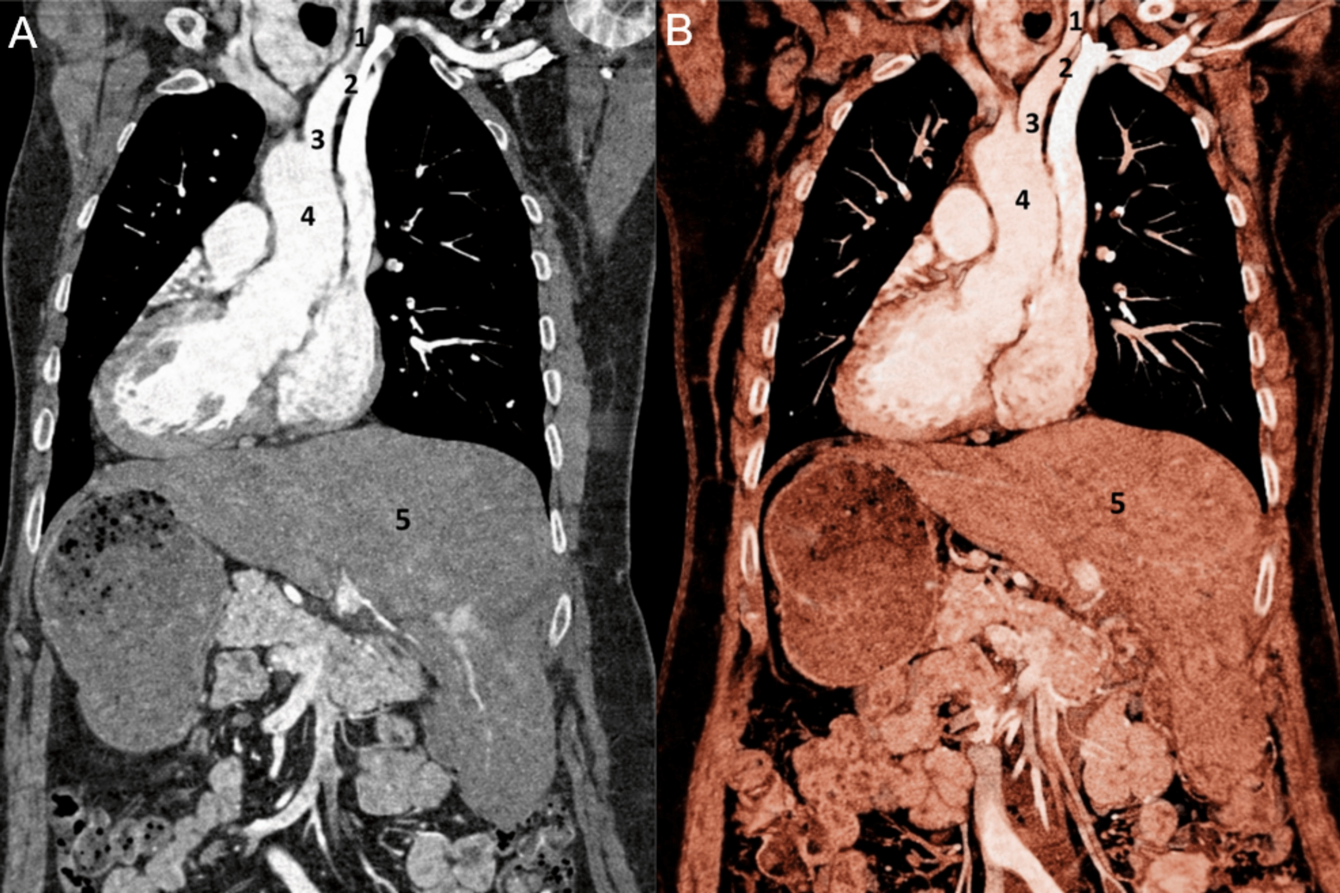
Thoracoabdominal computed tomography angiography (CTA) demonstrating the origin of the great vessels from the aortic arch and mirror-image visceral anatomy. (A) Coronal maximum intensity projection image. (B) Three-dimensional volume-rendered reconstruction. 1: left common carotid artery; 2: left subclavian artery; 3: left brachiocephalic trunk; 4: aortic arch; 5: liver
